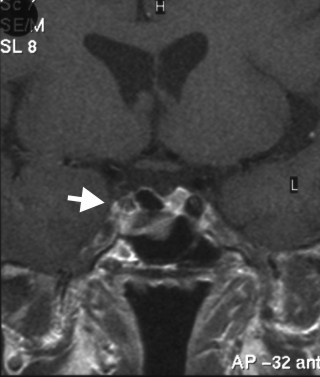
MRI of Pituitary – post operative.
